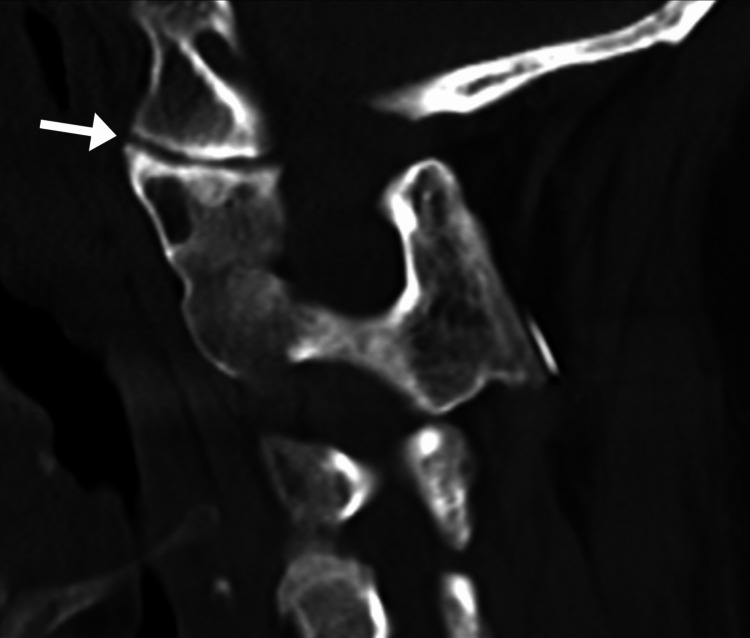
Sagittal computed tomography section through the left atlanto-occipital joint The joint is narrowed (arrow), consistent with mild degenerative changes (osteoarthritis).
